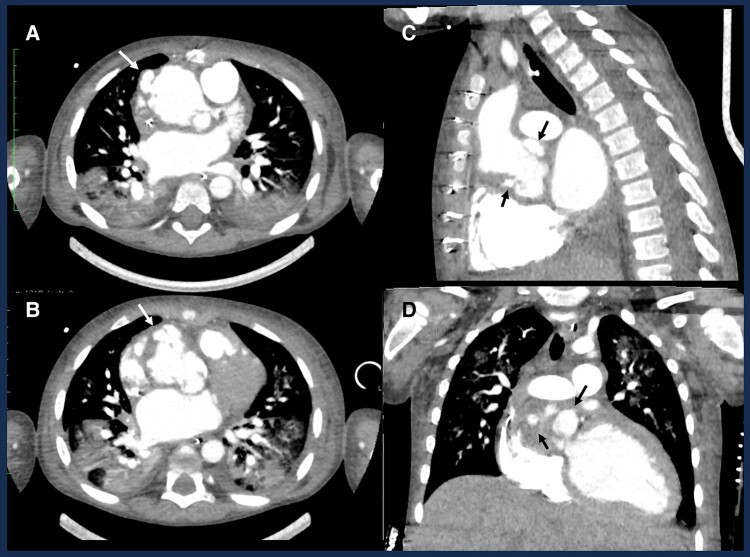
Chest computed tomography scan showing several pseudoaneurysm and abscess (arrows) regarding the aortic root and ascending aorta on axial (*A* and *B*), sagittal (*C*), and coronal (*D*) views.
